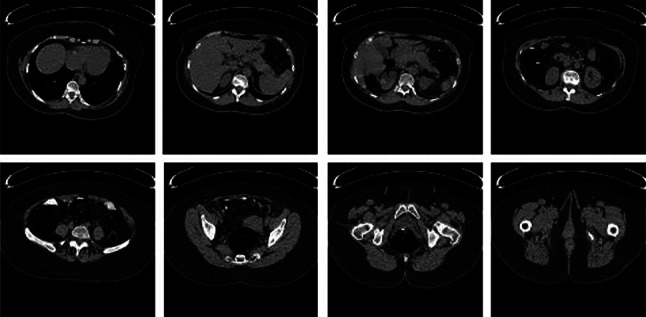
Sample images from each class of TCIA-CT database
